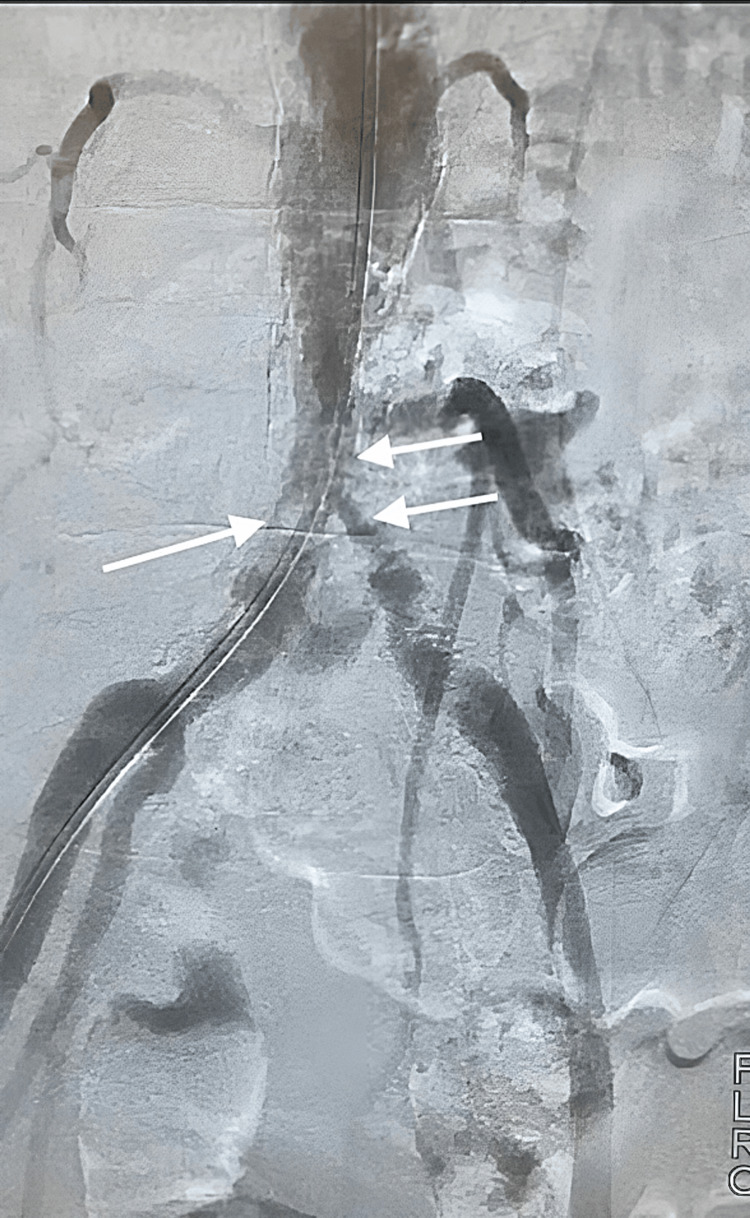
Pre-operative computed tomography (CT) angiography displaying severely calcified arteries with moderate stenosis of distal aorta and severe stenosis of bilateral common iliac arteries.
